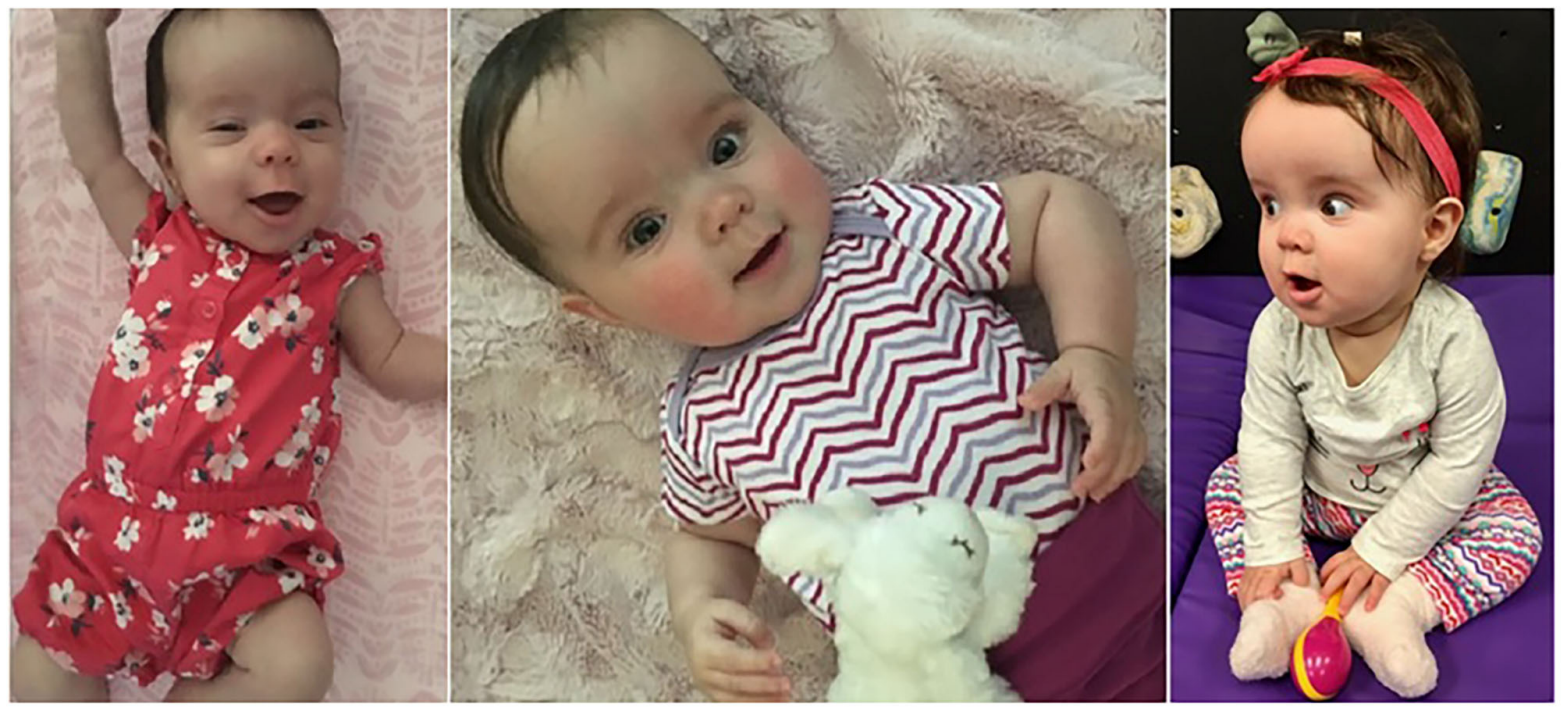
A patient diagnosed with phosphatase and tensin homolog (PTEN)-hamartoma tumor syndrome. She was brought to medical attention shortly after birth for concerns of macrocephaly and hypotonia. Her brain MRI was normal. At 14 months, her fronto-occipital circumference (FOC) was 52.6 cm (+5.38 SD) and 56.4 cm (+5.06 SD) at 35 months. She has been receiving physical therapy since age 6 months due to hypotonia and also speech therapy for expressive language delays. FOC > 3 SD *even as isolated finding* is suspicious of PTEN-hamartoma tumor syndrome. Due to the increased risk for malignancy (see text and Table 2), she will undergo childhood cancer screening (thyroid carcinoma) and later adult cancer screening (breast, thyroid, endometrial, and colon). Developmental delays are common in this syndrome.
